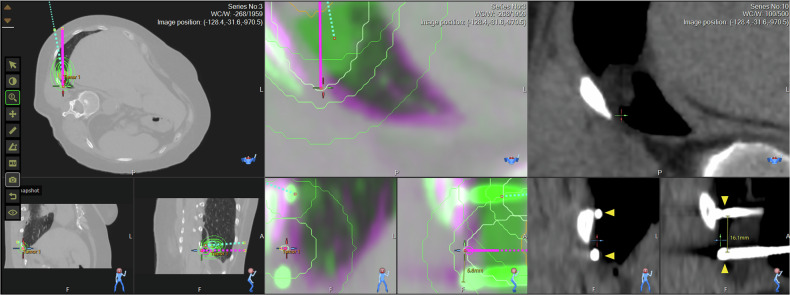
‘Chopstick’ plan and targeting accuracy. A 63-year-old woman undergoing cryoablation of a subpleural right lower-lobe colorectal metastasis. Left: Planning CT showing intended cryoprobe trajectories and manufacturer-supplied isotherms. Centre: Fused planning and verification CT demonstrating assessment and measurement of targeting accuracy between planned (magenta) and actual (green) needle paths. Right: Verification CT showing final cryoprobe positions in a “chopstick” configuration (yellow arrowheads), with probes flanking the target lesion and satisfactory for ablation
